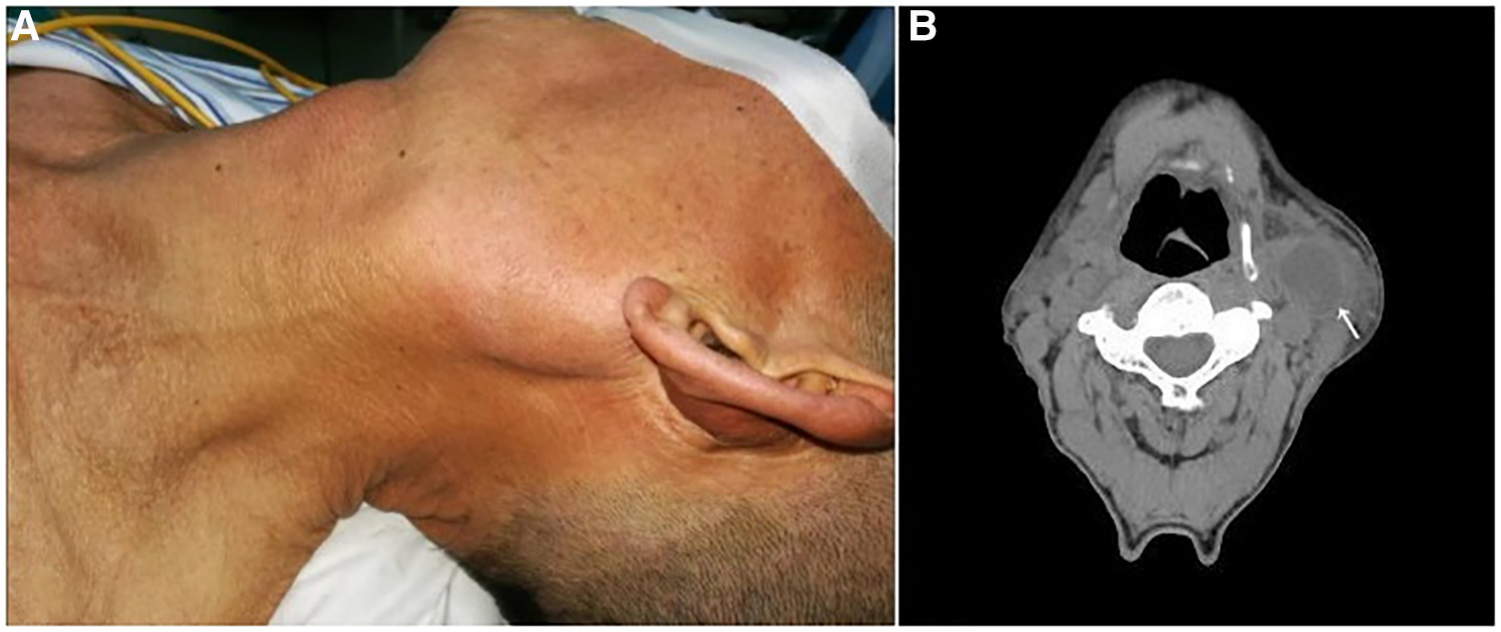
(A) The general view before resection. (B) The CT image of the branchial cleft cyst.
